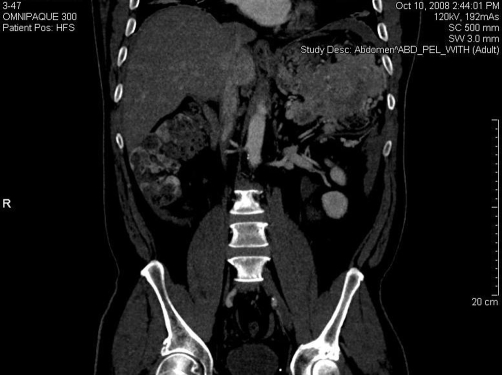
Computed tomography (CT) of the abdomen and pelvis. A large 4.7 centimeter (cm) lobulated hypodense mass is shown in the region of the porta hepatis likely secondary to the tumor mass.
